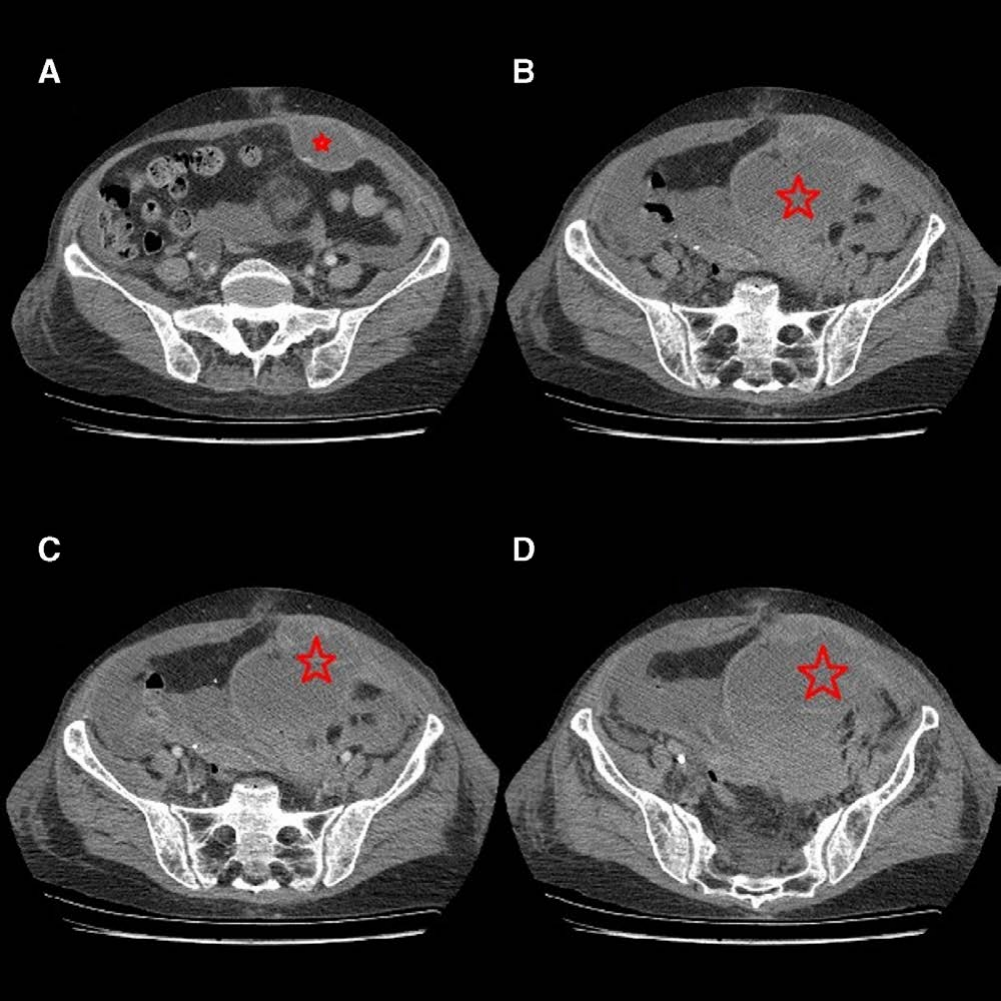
Cross-sectional CT scan of the abdomen showing the sheath hematoma. Star: Left rectus sheath hematoma. (A) No intraperitoneal extension. Note the intraperitoneal extension in B–D. CT = computed tomography.
